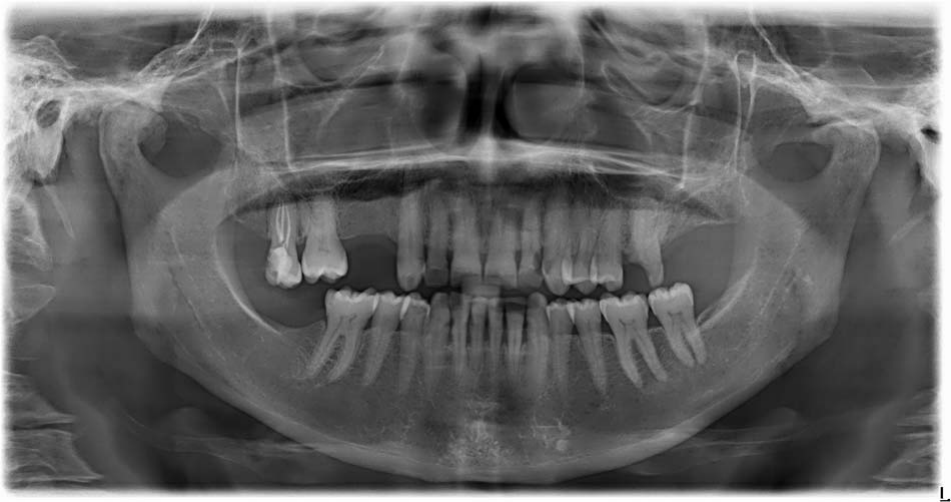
Panoramic radiograph confirms the findings.
